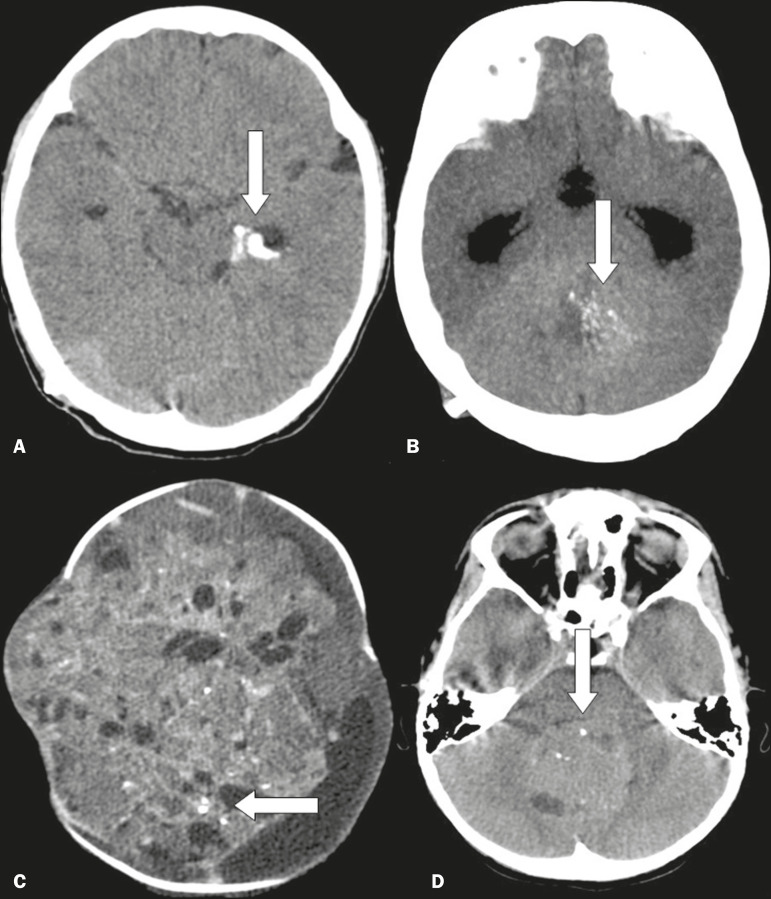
Unenhanced axial cranial CT scans, with soft-tissue window settings, showing coarse, peripheral calcifications (arrow), together with a temporal cystic lesion, in a case of ganglioglioma (A), multiple, small confluent calcifications (arrow) in an expansive lesion in the posterior fossa, in a case of ependymoma (B), small, sparse calcifications (arrows) in a voluminous expansive supratentorial lesion in a child with a pineal gland tumor (C), and small sparse calcifications (arrow) in an expansive lesion in the posterior fossa in a case of medulloblastoma (D).
